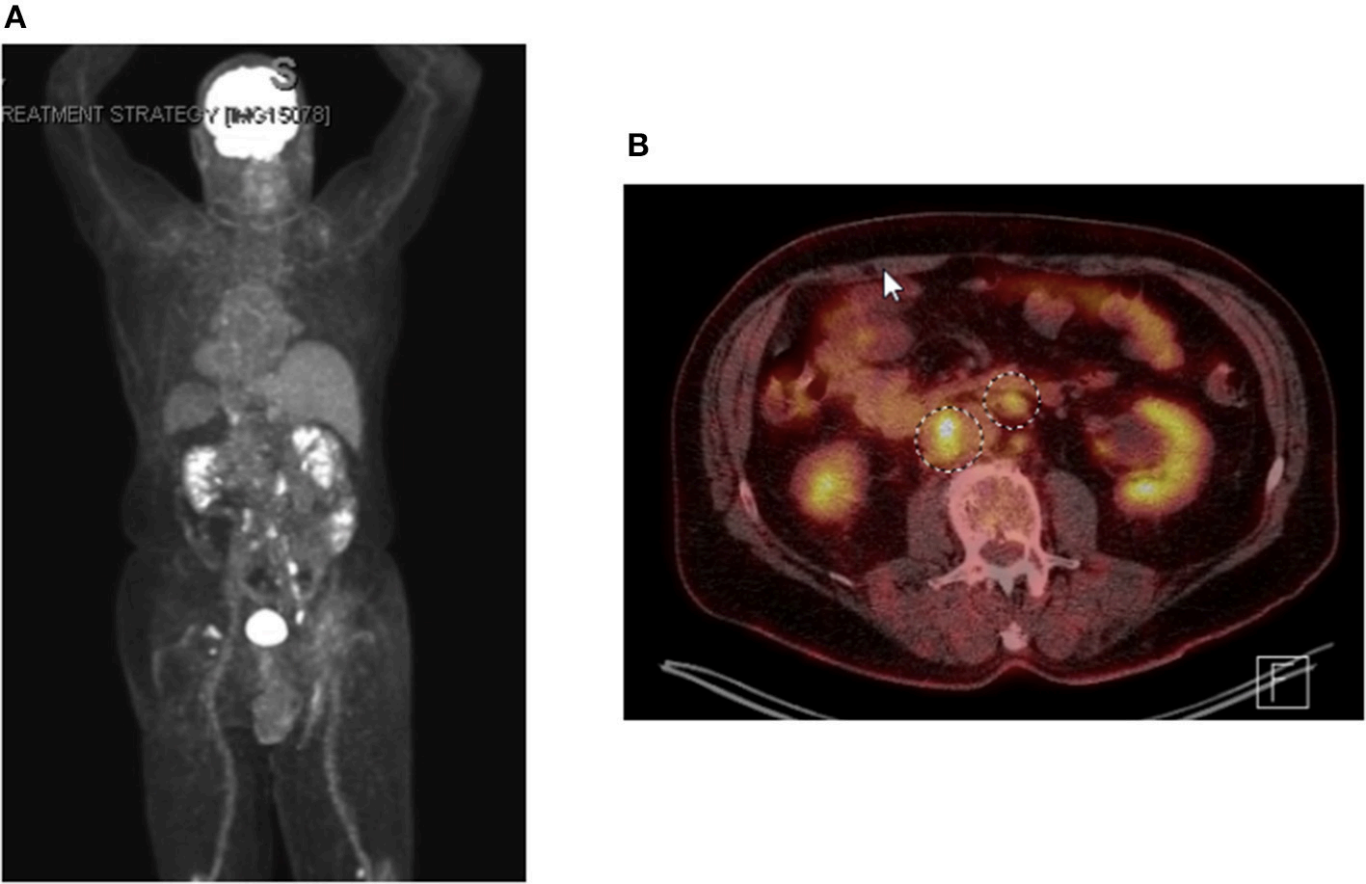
PET-imaging demonstrating metastatic dissemination of Merkel cell carcinoma. (A) whole body positronic imaging showing bilateral pelvic and retroperitoneal SUV avidity consistent with metastatic disease. (B) representative fused PET CT-scan axial imaging showing SUV avid retroperitoneal adenopathy.
